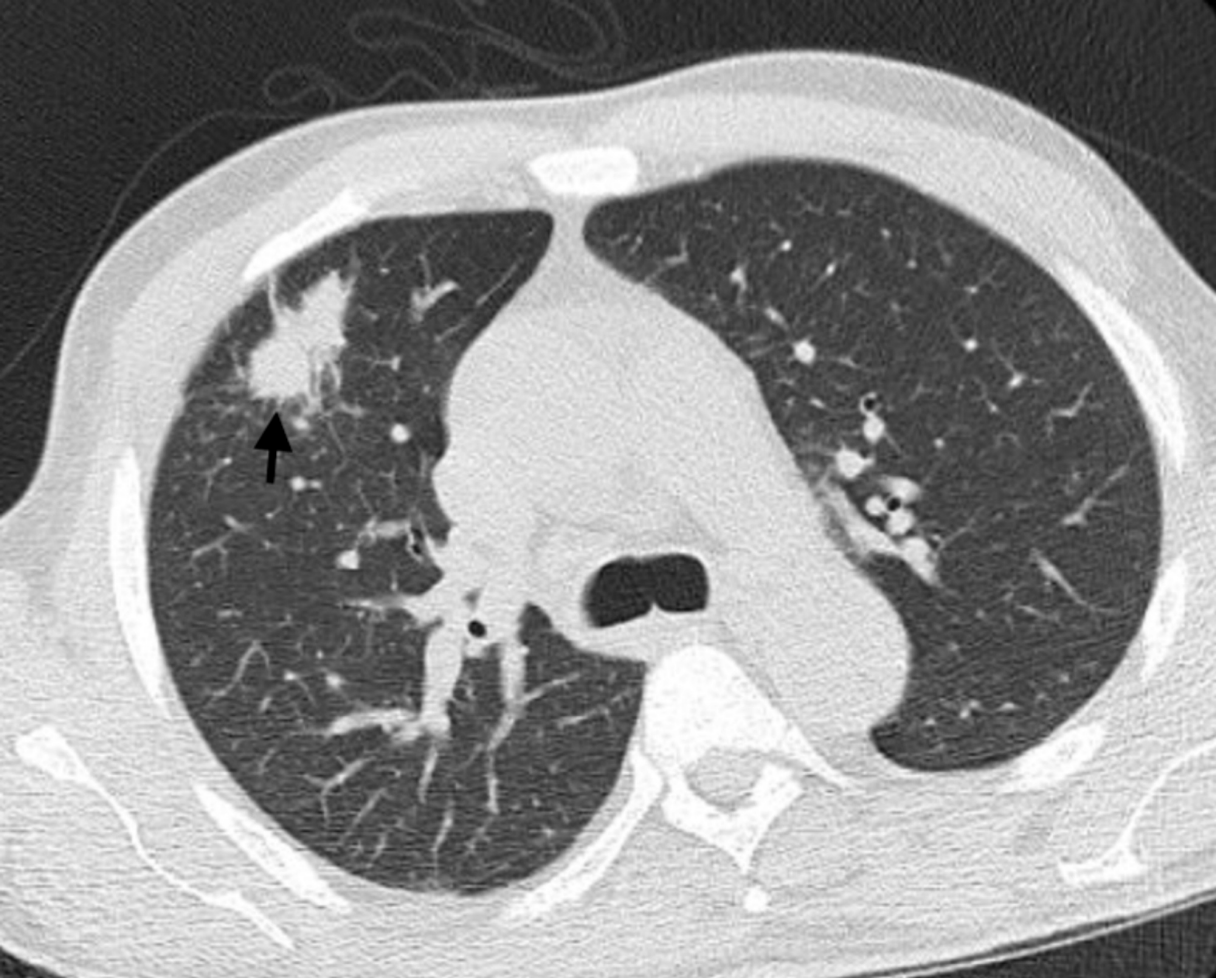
CT thorax showing pulmonary nodule (arrow)
